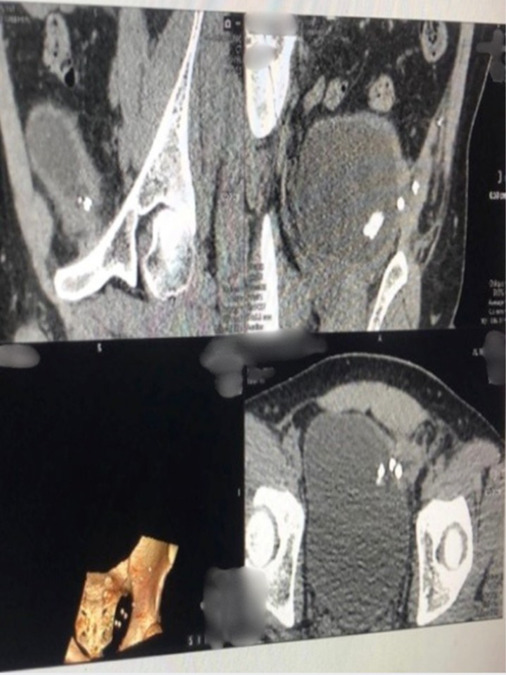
Contract enhanced CT Pelvis sagittal, coronal, axial and 3D reformats showing hernial mesh projecting into urinary bladder
